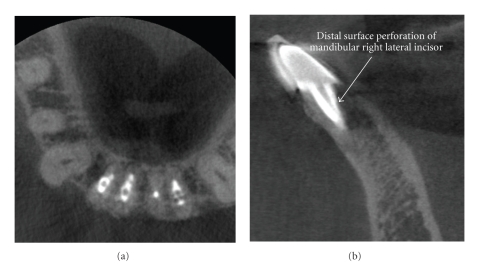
Iatrogenic perforative defects can be difficult to confirm by periapical radiography alone. This patient was referred for evaluation and possible endodontic revision of the mandibular right lateral incisor because of chronic sensitivity to occlusal forces. CBCT images ((a) axial, (b) sagittal) demonstrate a mid-root post perforation.
